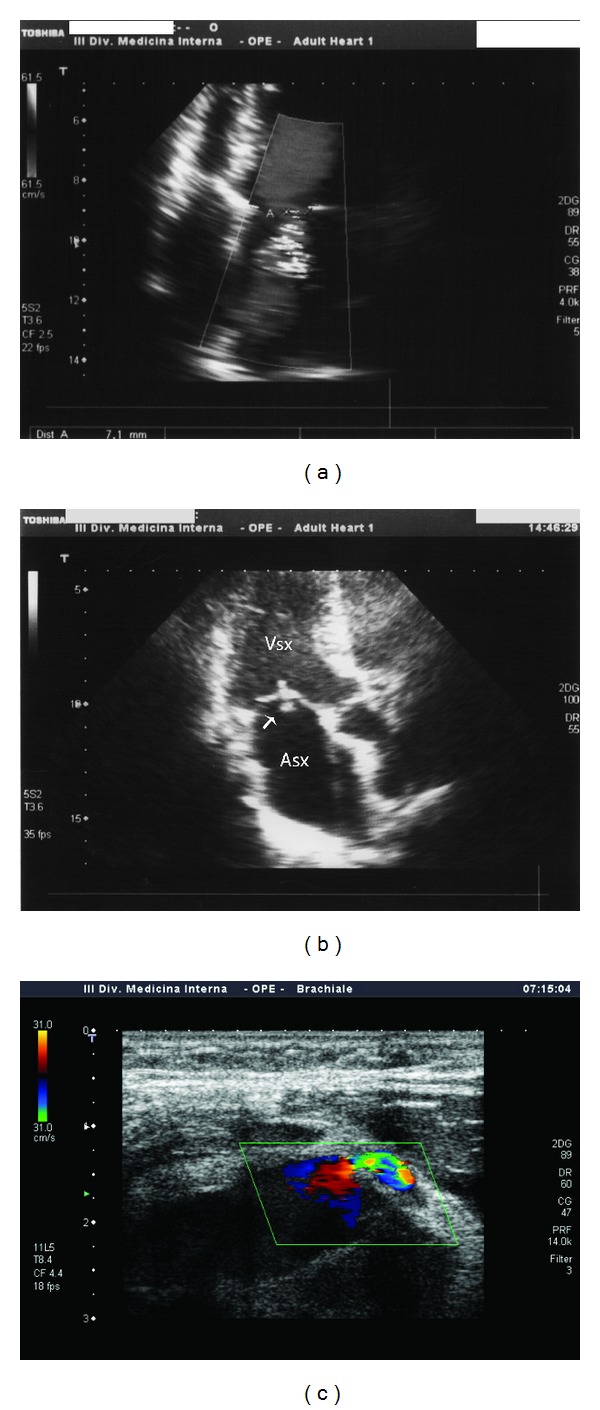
(a) Color Doppler imaging showing mild-to-moderate mitral regurgitation; (b) three-chamber apical view showing vegetation on distal third of mitral anterior leaflet; (c) echography of anterior aspect of right forearm showing color Doppler imaging of radial artery refueling perivascular blood collection.
